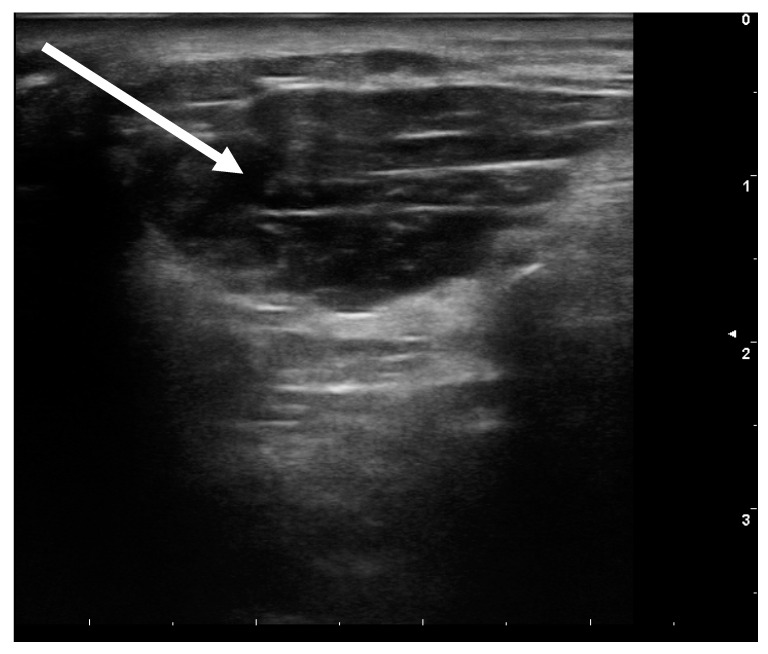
Ultrasound image of the levator scapulae muscle. Clearly visible are the four parts of the muscle and the change in morphology compared to the norm (notably rounded).
